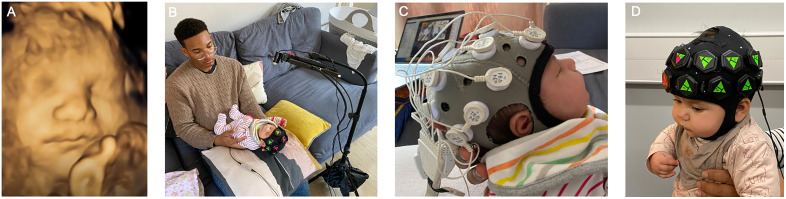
Panel A – 4D ultrasound scan at 34 weeks gestation; Panel B – 1-month-old infant wearing fNIRS headgear, supported by a researcher while sleeping in the home setup; Panel C – 1-month-old infant wearing EEG headgear supported by a researcher while sleeping in the home setup; and Panel D – and 5-month-old infant wearing fNIRS headgear at the research lab visit.
